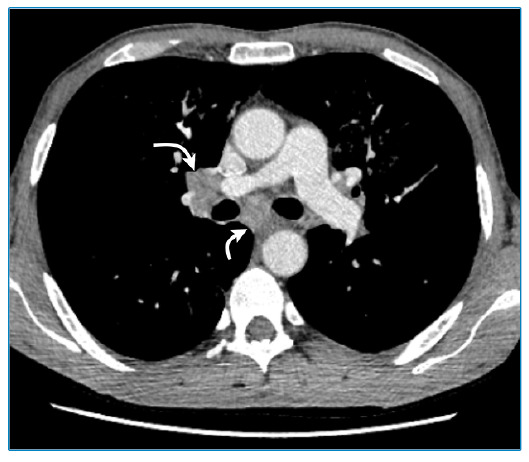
Thoracic computed tomography showing mediastinal lymphadenopathy (curved arrows).
